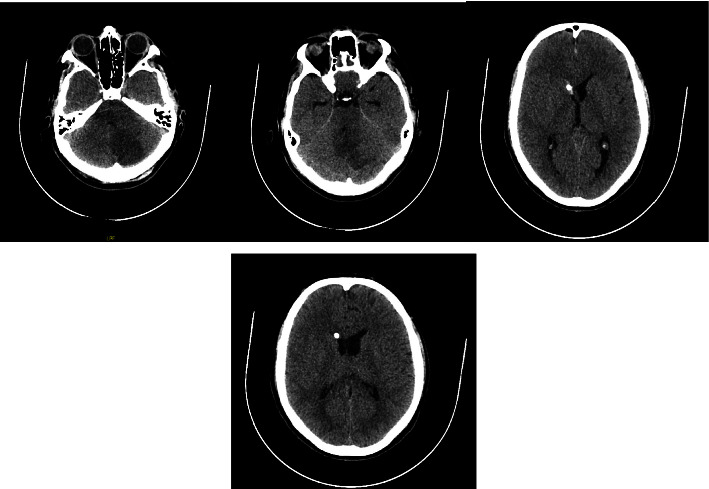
Simple control computed tomography of the skull. (a). Established infarction in the left cerebellar hemisphere. (b). Ventricular and cisternal systems with normal shape, size, and position. (c). A ventricular bypass catheter enters through the right frontal region, with the distal end in the frontal horn of the right lateral ventricle. (d). Decrease in size of the right ventricular system compared with the contralateral side.
